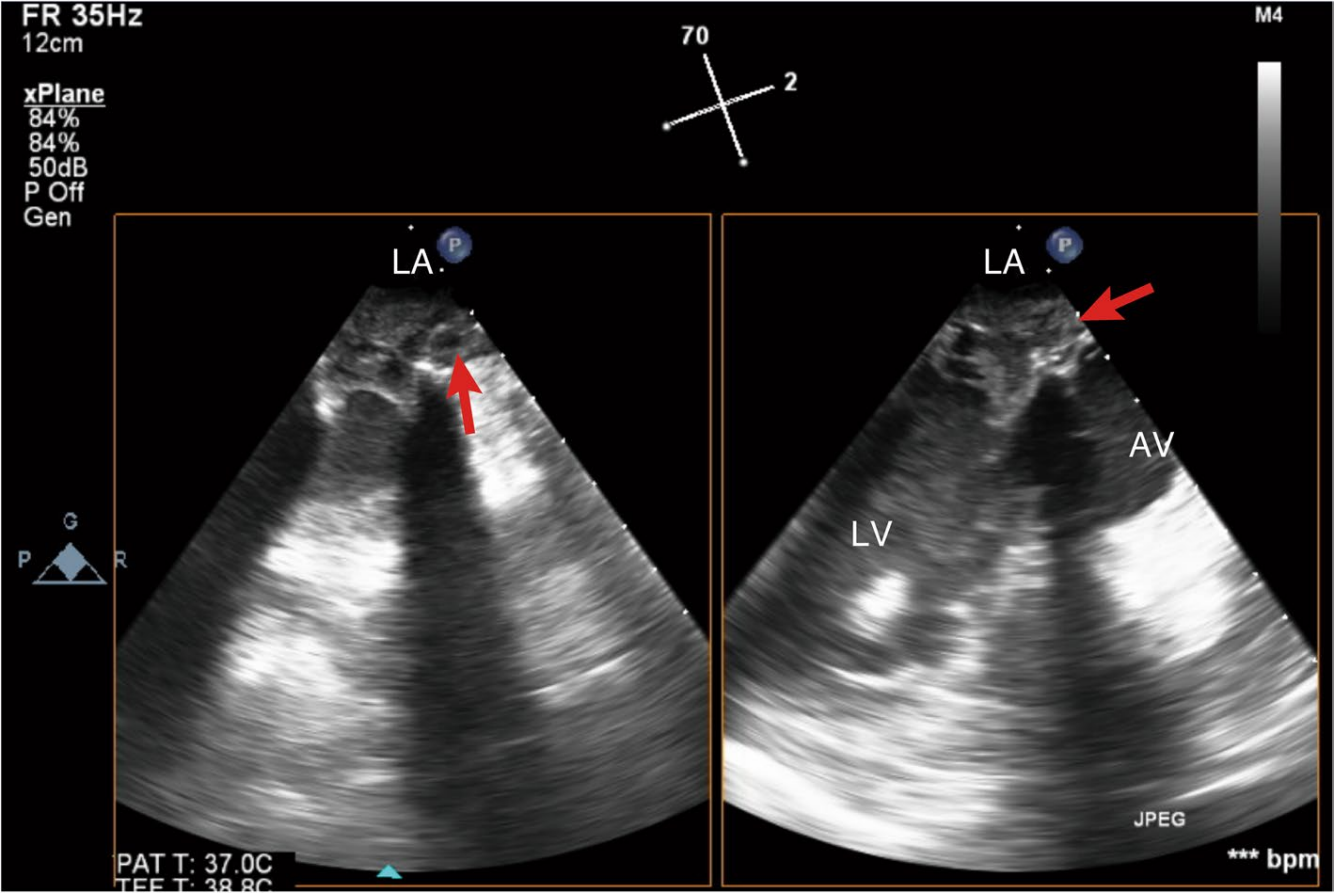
Mid-esophageal mitral commissural view (left) and long axis view (right). The prosthetic mitral valve occluded by a left atrial thrombus (arrow) was observed on postoperative day 1, after the initial mitral valve replacement by transesophageal echocardiography performed in the ICU. AV, aortic valve; LA, left atrium; LV, left ventricle
